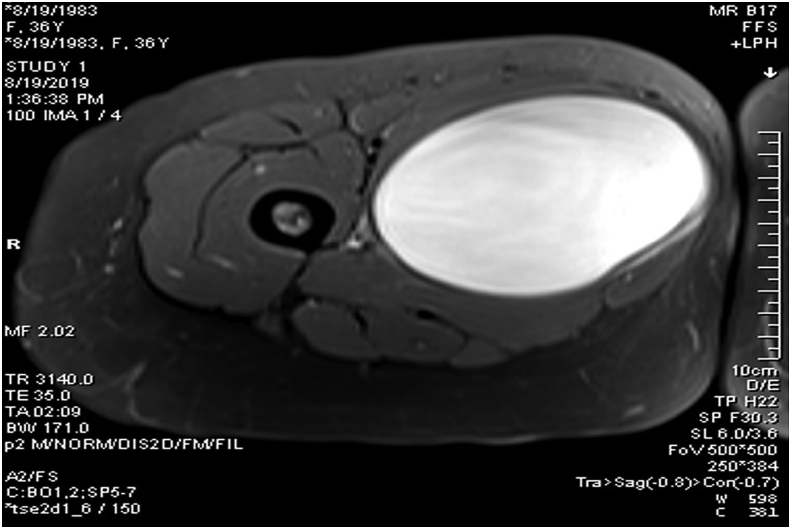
Transverse plane by MRI scan shows a cystic mass in the right thigh closed to the deep femoral artery, it was characterized by a low single intensity in T1-weighted scan and high signal intensity in T2-weighted scan.
